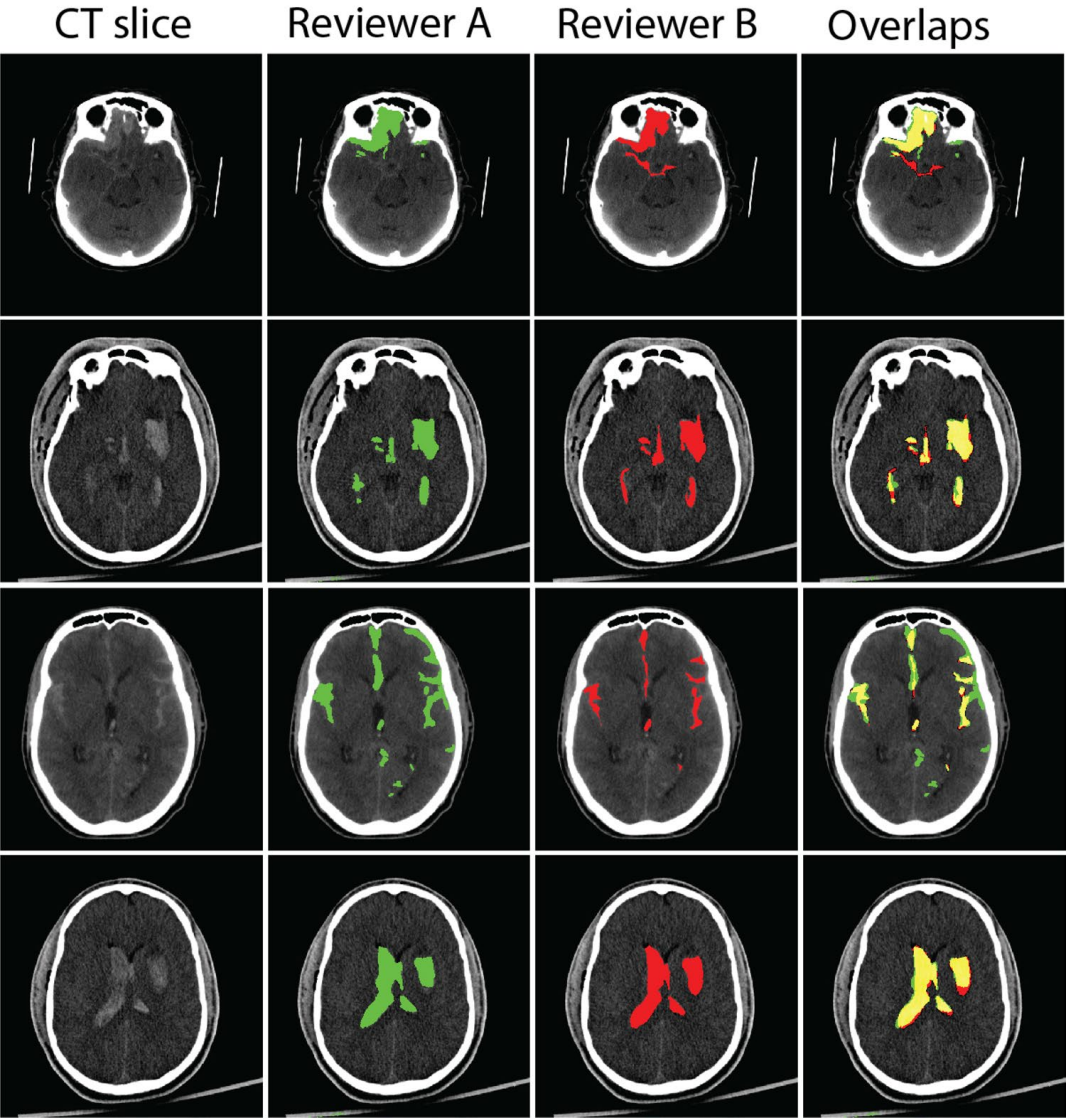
Comparison of human error in hematoma segmentation between two reviewers. Each row represents a selected slice from the peer-reviewed dataset. The first column shows the contrast-adjusted CT slices with a window level of [0–140] HU. The middle columns display the manual segmentations by Reviewer A (in green) and Reviewer B (in red), respectively. The last column presents the overlap of annotations from both reviewers, with the intersecting regions shown in yellow and the non-overlapping annotations retaining their original colors (green for Reviewer A and red for Reviewer B)
